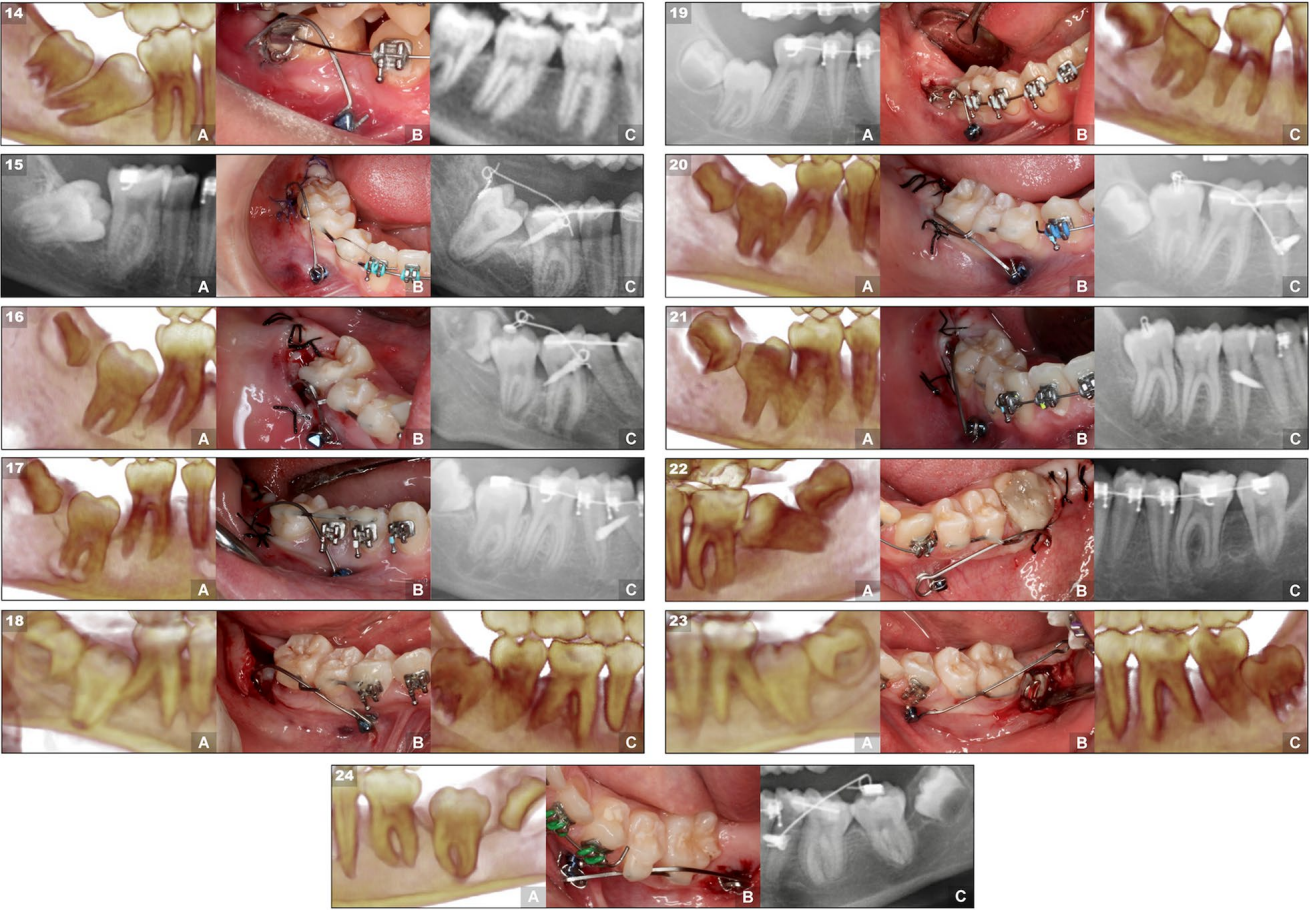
Case series of mandibular M2s treated with the miniscrew-supported pole technique. The 11 cases (from 14 to 24) are organized according to their angulation, from mesial to distal, showing first the rights and then the left ones. Each case is composed of three images that represent three moments during the treatment: a pretreatment radiograph showing the impacted or retained lower molar. b molar with the pole technique surgery carried out. c radiograph showing the final result with the molar in a correct position and angulation
